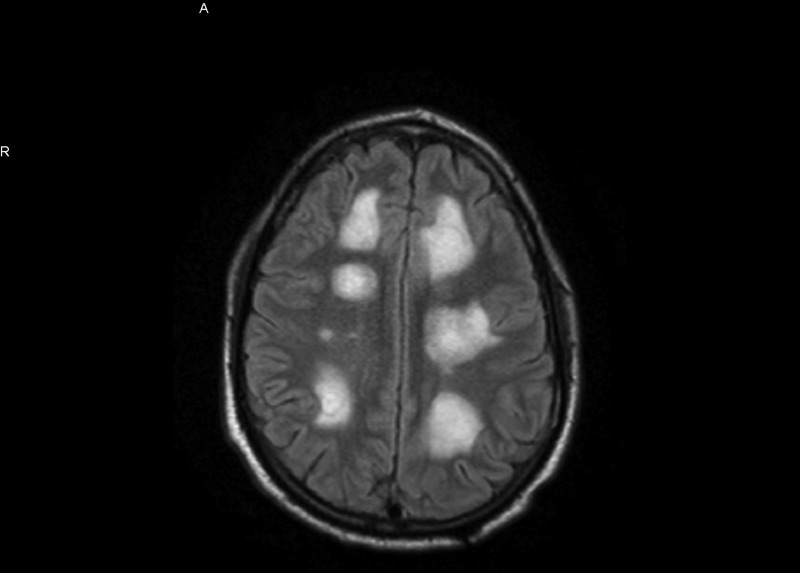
MRI with contrast after corticosteroid treatment shows increased size of the lesions. Bilateral white lesions seen in MRI
